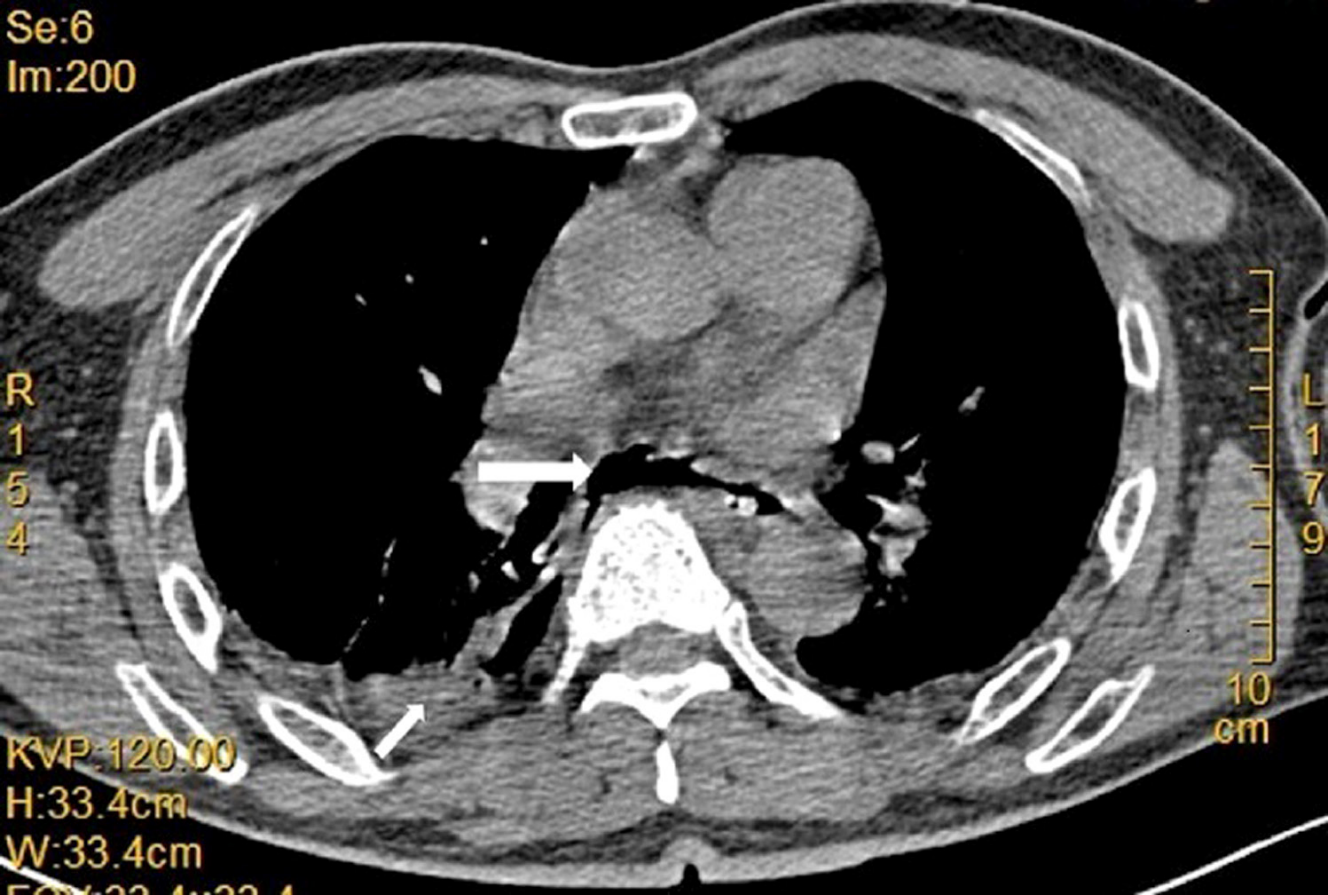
Computed tomography scan identified right-sided hydropneumothorax and pneumomediastinum.
